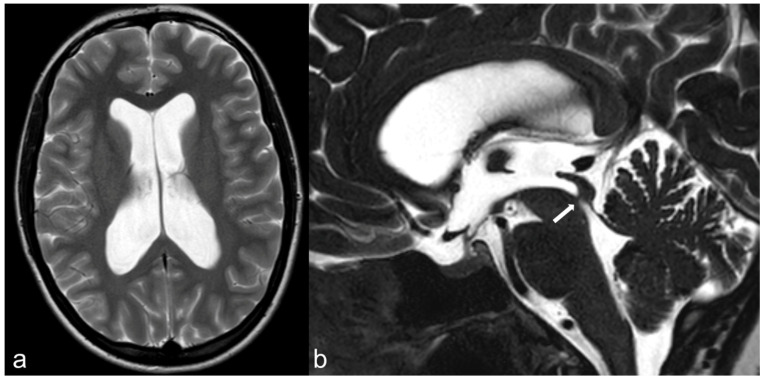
26-year-old female patient presenting with long-standing headache, unresponsive to medical therapy. MRI reveals obstructive supratentorial hydrocephalus. (a) Axial T2-weighted (T2-w.) turbo spin echo (TSE) image shows enlargement of the lateral ventricles. No signs of transependymal edema are apparent. (b). 3D CISS depicts a subtle hypointense membrane (arrow) located at the level of the cerebral aqueduct. Findings are consistent with aqueductal web. The patient subsequently underwent endoscopic third ventriculostomy.
